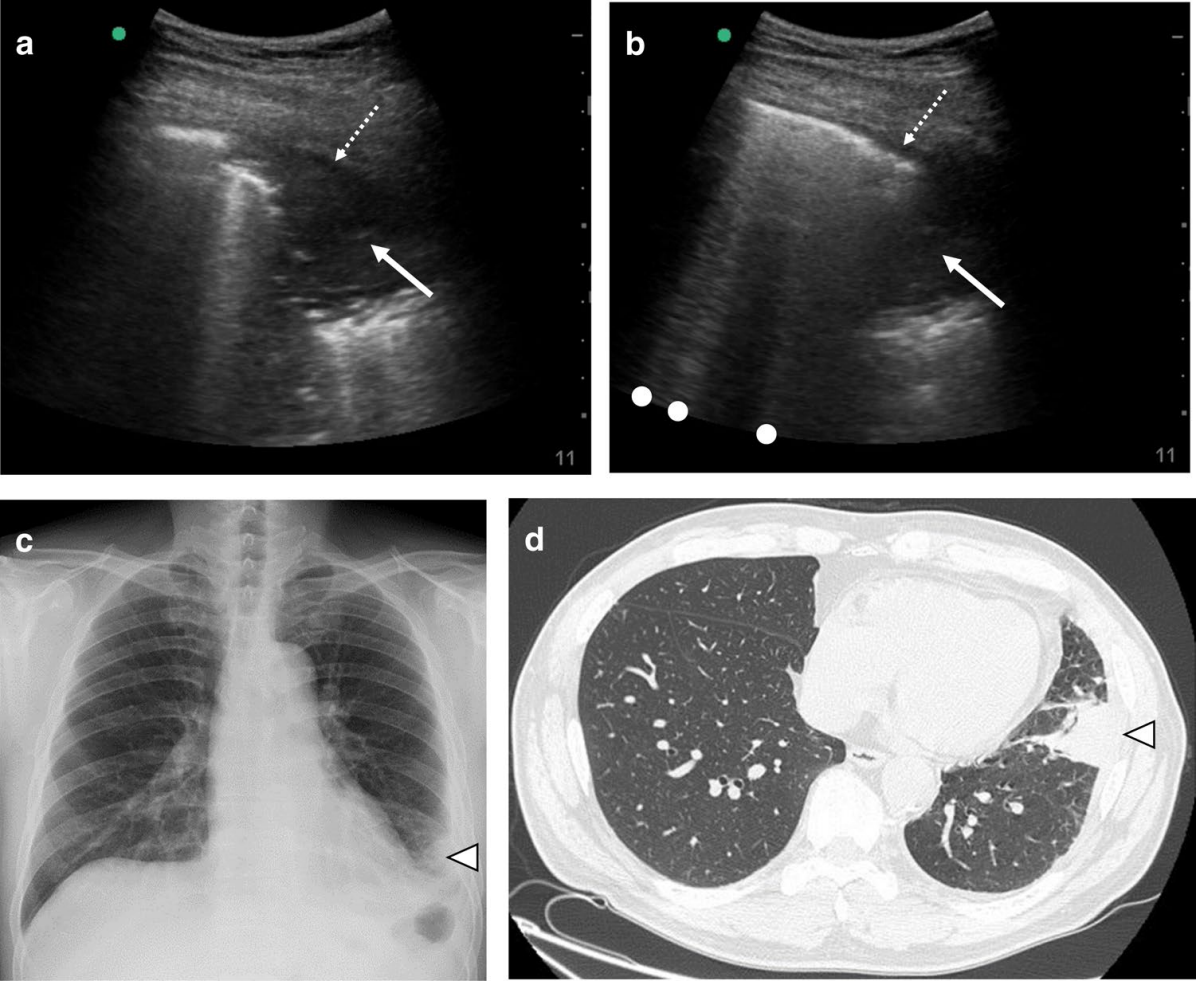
Lung ultrasound images (a, b), a chest X-ray image (c), and a chest computed tomography (CT) image (d) from a patient who presented with fever and left pleural pain. Lung ultrasound revealed sonographic consolidation (arrows) with slight pleural effusion (dotted arrows) in the left lower lateral chest, which corresponded to the site of the pleural pain. Multiple B-lines (dots) were observed around the site of sonographic consolidation. Chest X-ray and chest CT also revealed the consolidation (arrowheads). He was finally diagnosed with bacterial pneumonia
